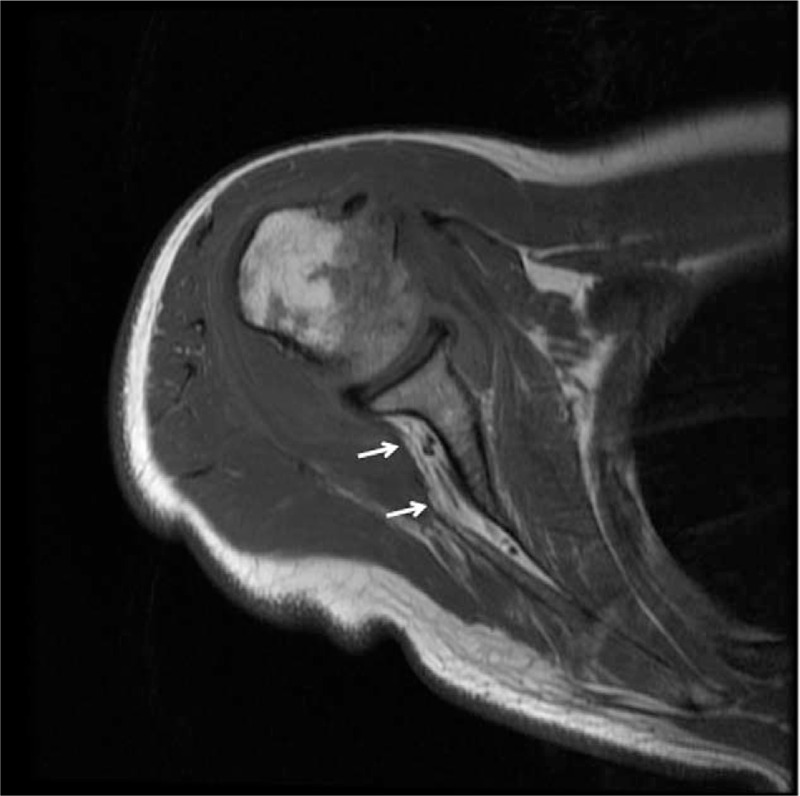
Axial T2-weighted magnetic resonance images of the right shoulder demonstrating fatty atrophy of the infraspinatus muscle (arrows).
